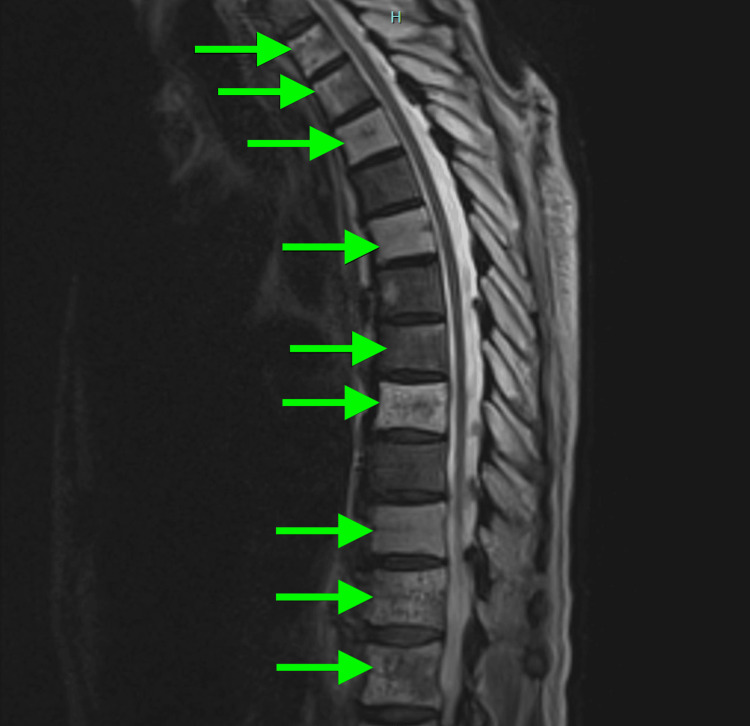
Emphysematous osteomyelitis of the thoracic spine on MRI. Sagittal T1-weighted MRI of the thoracic spine in an 80-year-old Caucasian man presenting with worsening generalized weakness, dyspnea, and tachycardia, showing heterogeneous appearance of the bone marrow in multiple vertebral bodies, including T1, T2, T3, T5, T7, T8, T10, T11, and T12, concerning for emphysematous osteomyelitis.
